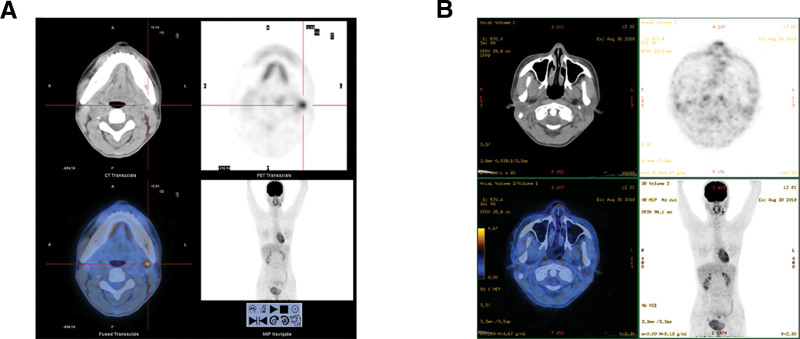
Images of whole-body ^18^F-FDG positron emission tomography-computed tomography performed during the initial clinic visit. (A) Enlarged submandibular lymph nodes were identified (the maximum diameter was about 1.2 cm). (B) No primary tumor was found in the body, especially in the nasal cavity.
